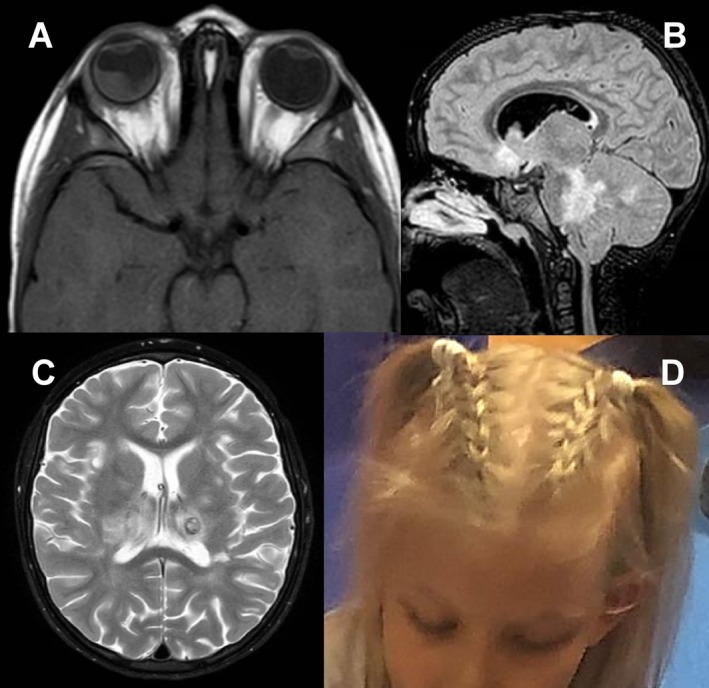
Magnetic resonance imaging revealing: (A) right optic neuritis (hyperintensity on T2); (B) lesions in both supra‐ and infratentorial regions with mass effect causing tonsillar herniation and obstructive hydrocephalus (hyperintense lesions on T2‐FLAIR) 3 months after onset; (C) hemorrhagic lesions in the thalamus, nucleo‐capsular regions, and pons (on T2‐FLAIR) were noted at 9.6 years. (D) Patient's hair and eyebrows with a typical silvery‐gray color.
